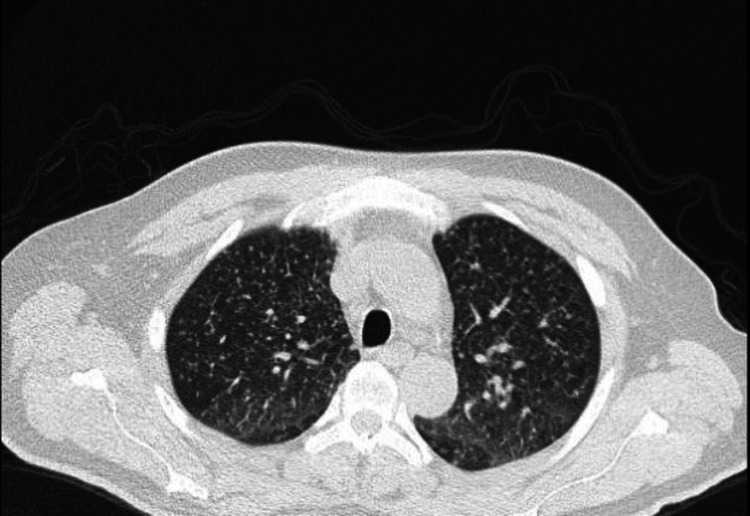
CT chest with contrast demonstrating extensive random miliary nodules bilaterally with an upper lobe predominance.
